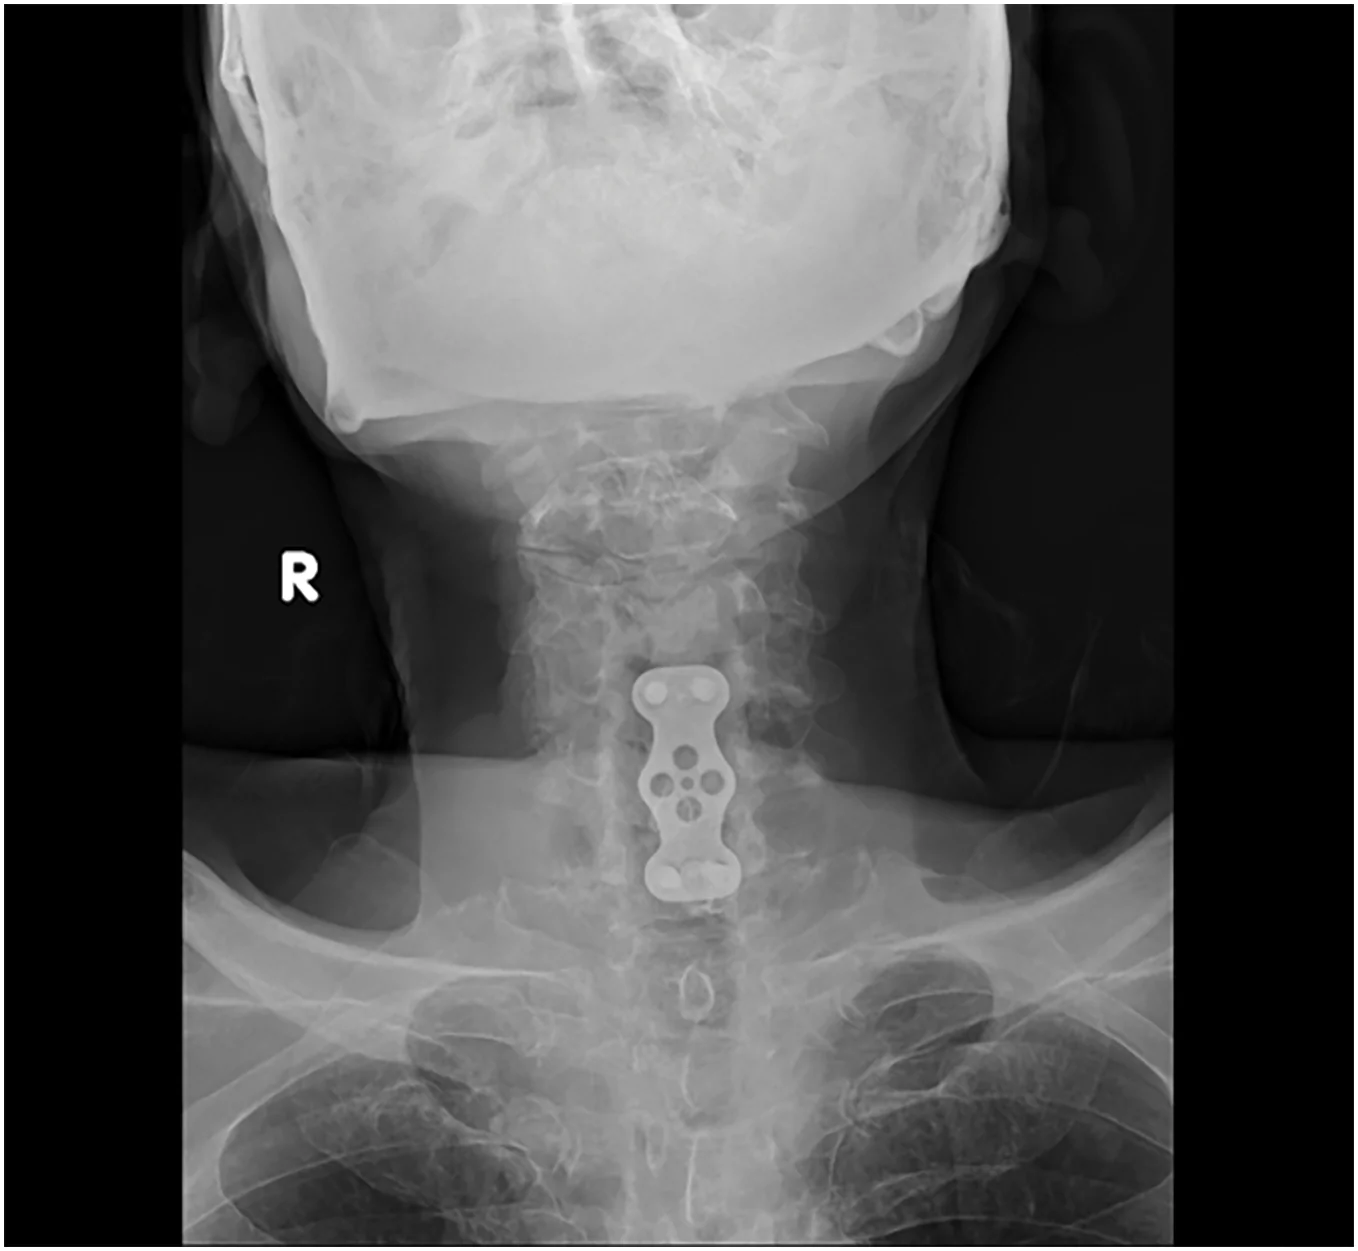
Radiographic examination of the neck revealed a metallic plate fixed to the cervical spine.
